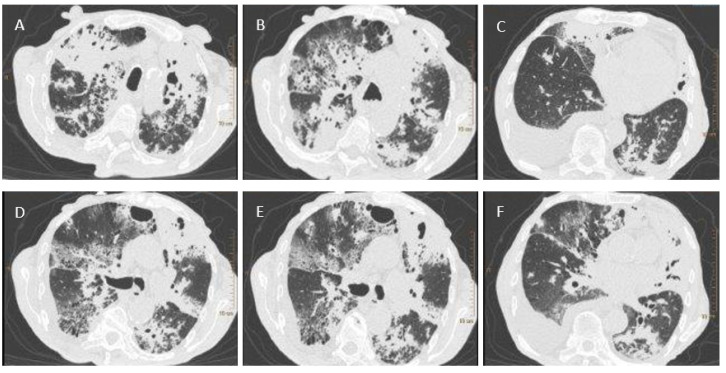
Figure 1. Chest CT scans. (A)—parenchymal-atelectatic lesions; thick-walled cavities filled with masses of tissue decay in the upper lobes; (B)—parenchymal-atelectatic lesions; cavities filled with tissue decay in segment 3 of the right and left lung, with the accompanying areas of tree-in-bud-appearance in segment 3 of the left lung and in the apex of segment 6 of the right and left lung; (C)—fluid in the pleural cavity; (D–F)—tree-in-bud-appearance in the apex of segment 6 of the right lung and in segments 6–9 of the left lung.
